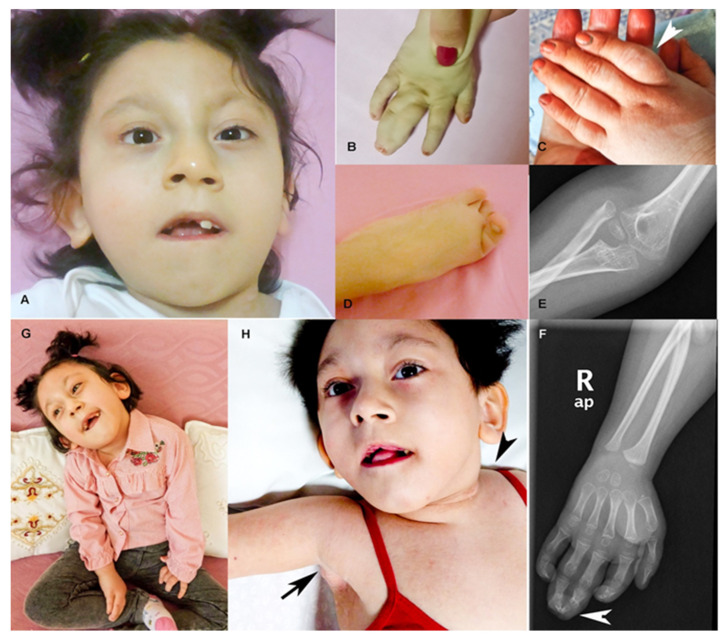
Pictures of patient 1. (A–D) Face, hand, and foot at age 9. Notice the asymmetry of the face, complete cutaneous syndactyly of the right second and third fingers, and overlapping toes. Thickened subcutaneous soft tissue on the proximal phalanges (prominently second finger) of the left hand (discoloration on the left hand due to traditional ‘red henna’). (E) Radial head dislocation. (F) Right hand radiograph showing distal phalangeal bone fusion of the third and fourth fingers at the age of 6. (G) Patient 1 at 9 years of age. Notice the generalized hypotonia. (H) Patient 1 at 6 years of age. Hyperemic, crusted skin lesions and focal dermal hypoplasia in her neck and axillary region and hypodontia are noticeable.
